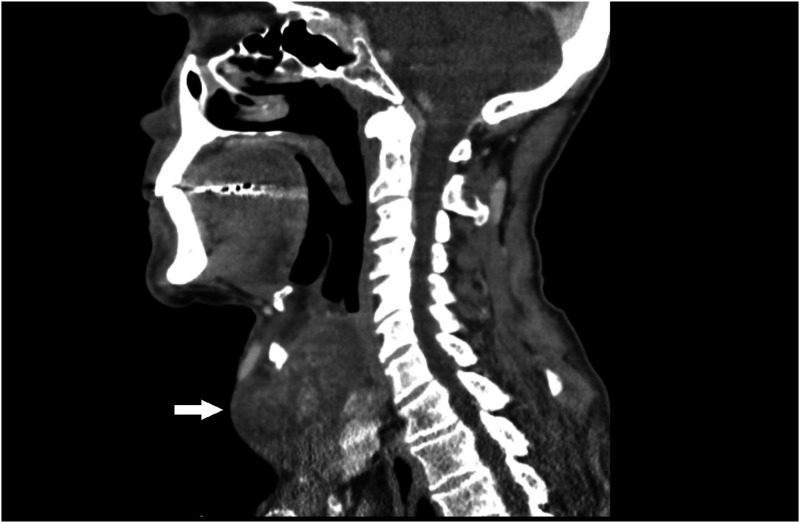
CT Soft Tissue of Neck to Show a Heterogeneous Enhancing Mass Measuring 6.3 x 5.8 x 4.1 cm (arrow pointed to the tumor).
